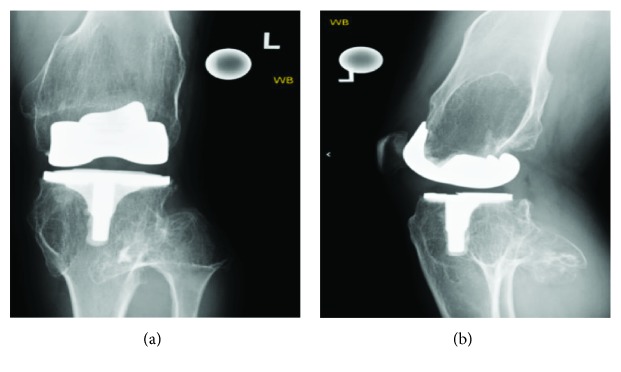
A two-month postoperative AP and lateral X-ray view of the patient's left knee displaying a Smith & Nephew posterior stabilized prosthesis with unchanged osteochondroma formation.
